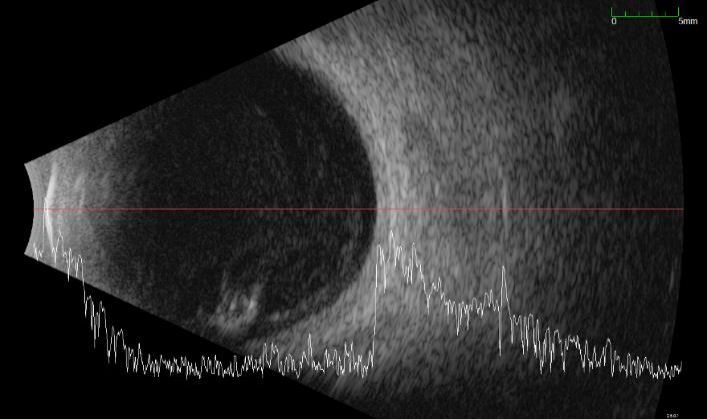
Right eye: ocular-orbital ultrasound, nasal transverse section, first day of examination
